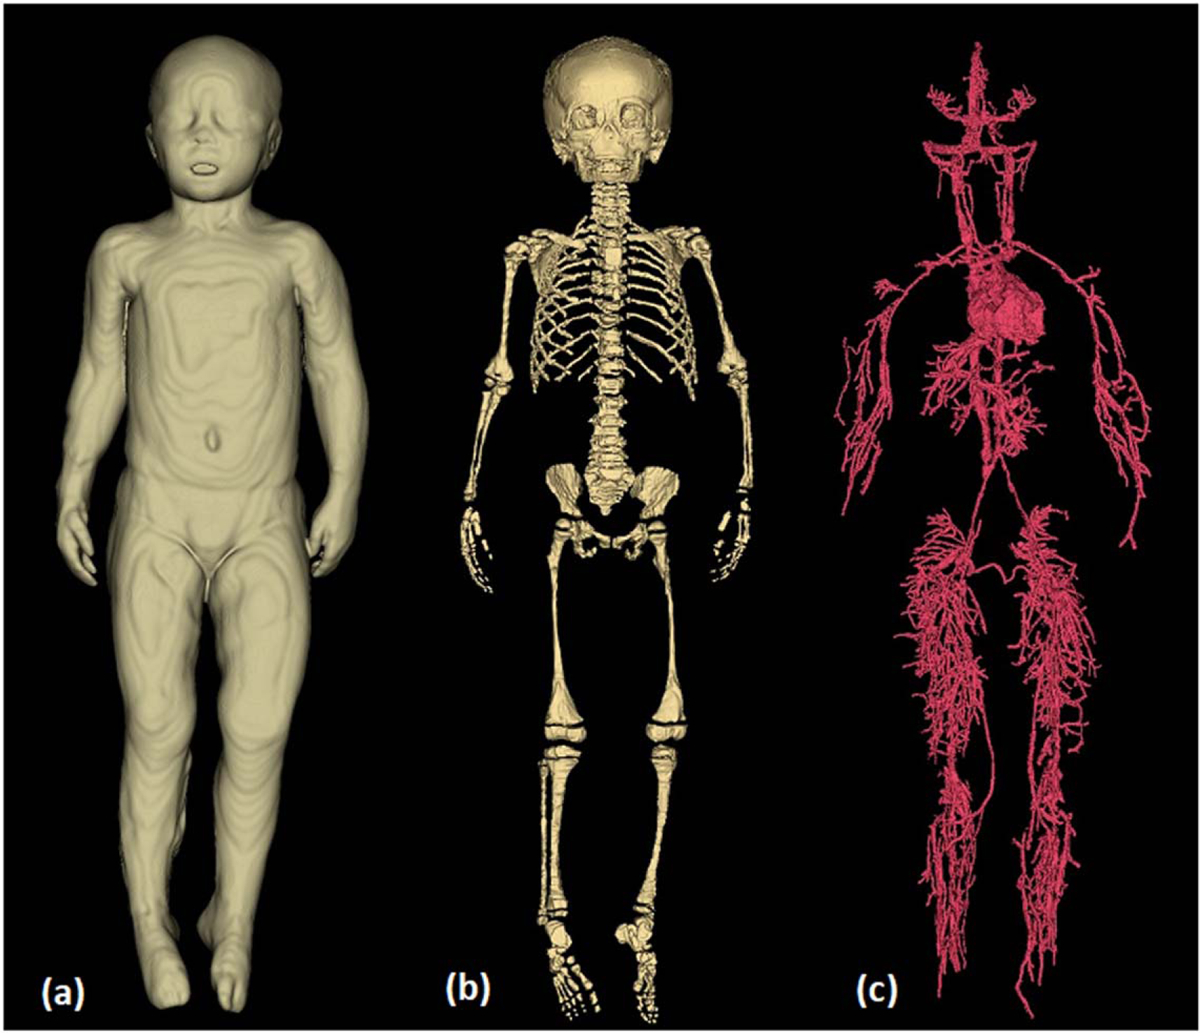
Whole body tissue segmentation for the development of a 3.5 year-old pediatric numerical model. (a) Whole body volume was segmented using a whole-body T1 MRI scan. (b) Whole-body bone segmentation. (c) Whole-body vessel segmentation, including extremities, head, and cardiac cavities based on T1 and IR images. MPRAGE and T2 Flair images were also used for the brain vessel segmentation.
